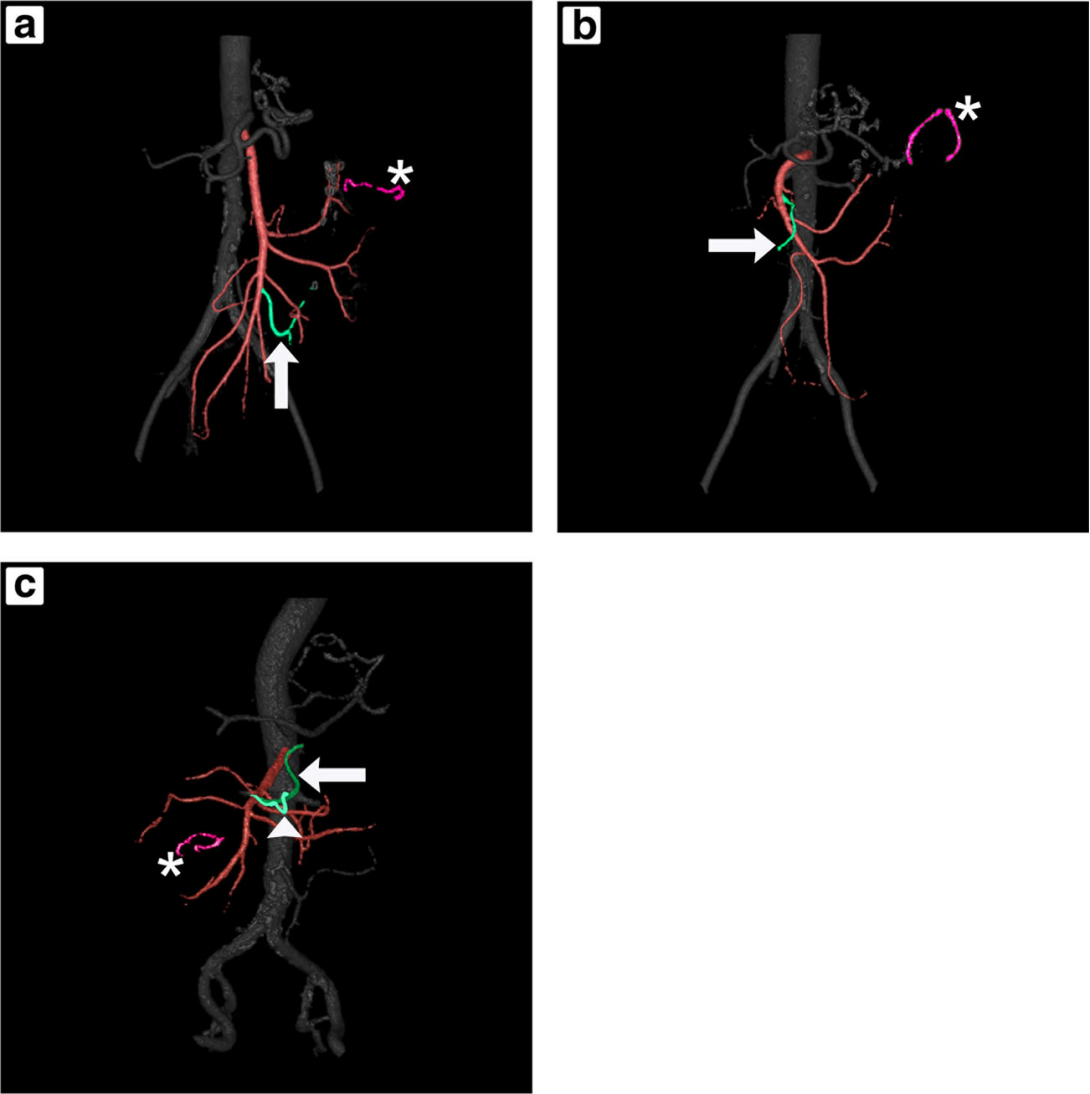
Anterior view of 3D CTA studies with presumed active internal hernias. Three hernia types were identified; common channel through enteroenterostomy defect (a), biliopancreatic limb through Petersen’s defect (b), and alimentary limb with enteroenterostomy through enteroenterostomy defect (c). Remarkable SMA branch configurations and enteroenterostomy staple line arrangements are indicated by an arrow(head) and an asterisk, respectively
